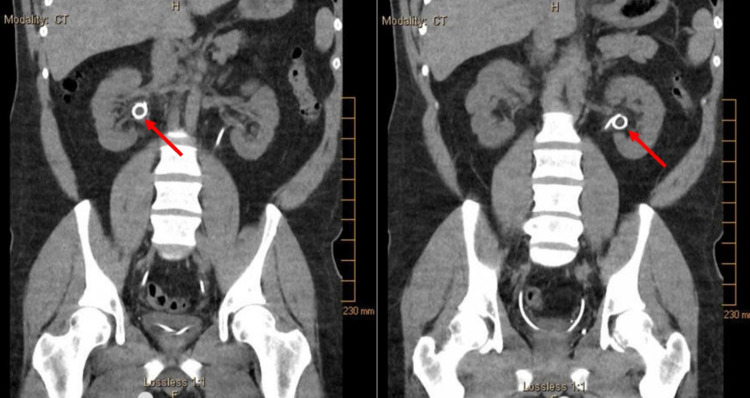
Abdominal and pelvic non-contrast CT with stent visualized in bilateral kidneys, ureteral obstructions, and hydronephrosis resolved.
